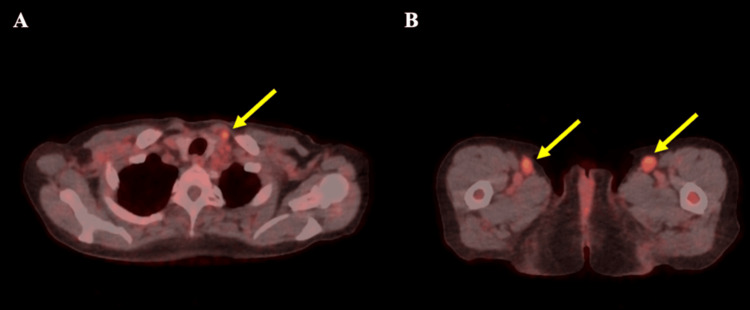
FDG-PET/CT images at diagnosis (A) Axial image showing a hypermetabolic supraclavicular lymph node (yellow arrow). (B) Axial image showing bilateral hypermetabolic inguinal lymph nodes (yellow arrows). PET-CT, positron emission tomography-computed tomography; FDG, fluorodeoxyglucose
